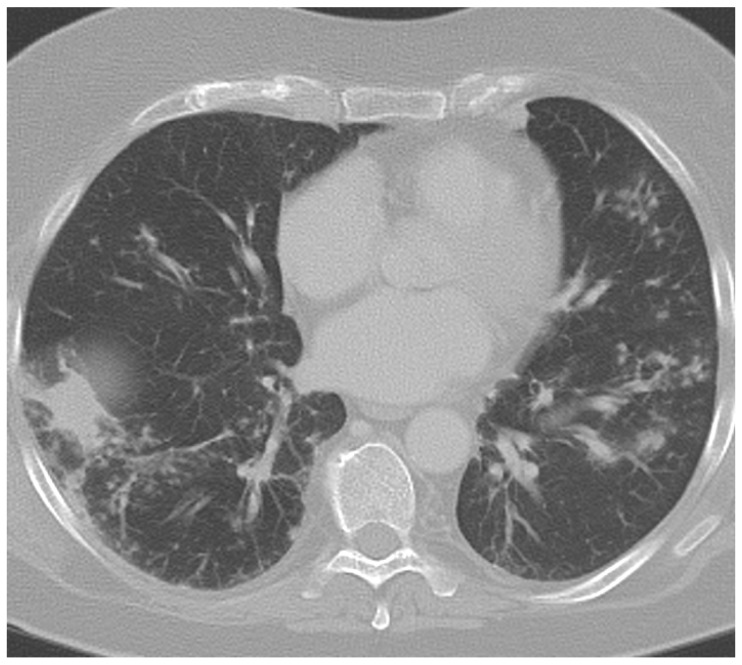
Chest CT in an elderly smoker with cardiovascular disease and atypical pneumonia compatible with *Legionella pneumophila*: segmental consolidation in the right lower lobe with surrounding ground-glass opacities, associated with small subpleural nodules diffusely distributed bilaterally.
